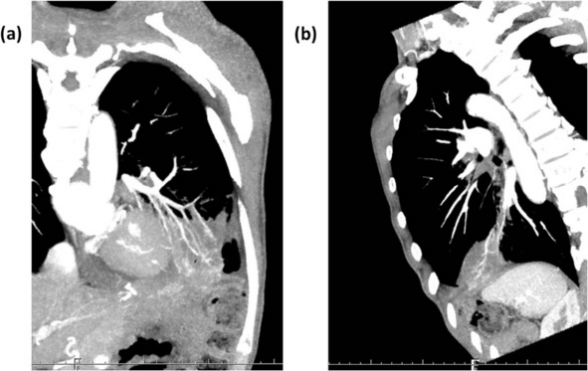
Preoperative chest CT of a coronal view and b sagittal view showed an irregular mass in the left lower lung, but no distinct fistula was detected
